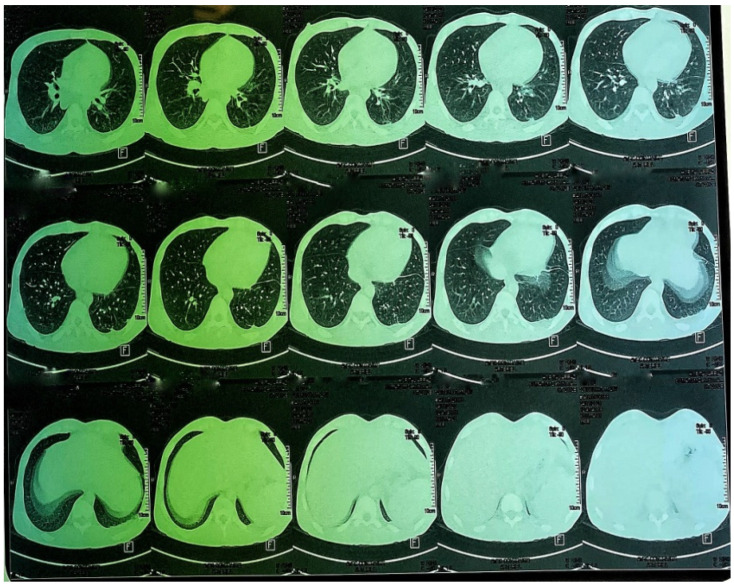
CT images of the thorax and abdomen.
